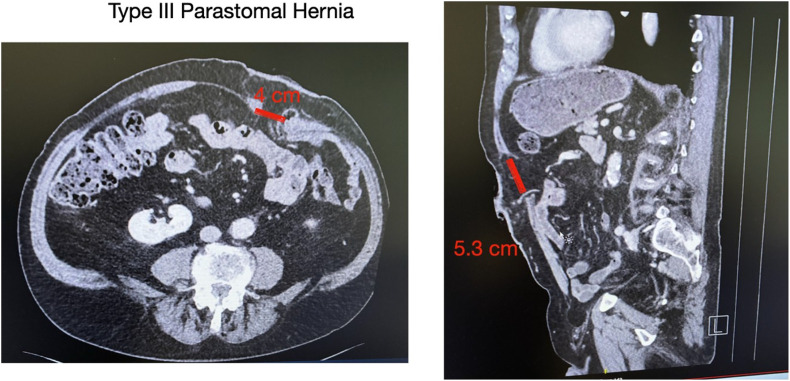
Preoperative CT imaging. Axial (left) and coronal (right) views showing a parastomal hernia measuring 4 cm in width and 5.3 cm in length. According to the European Hernia Society (EHS) classification, this corresponds to a type III parastomal hernia.
